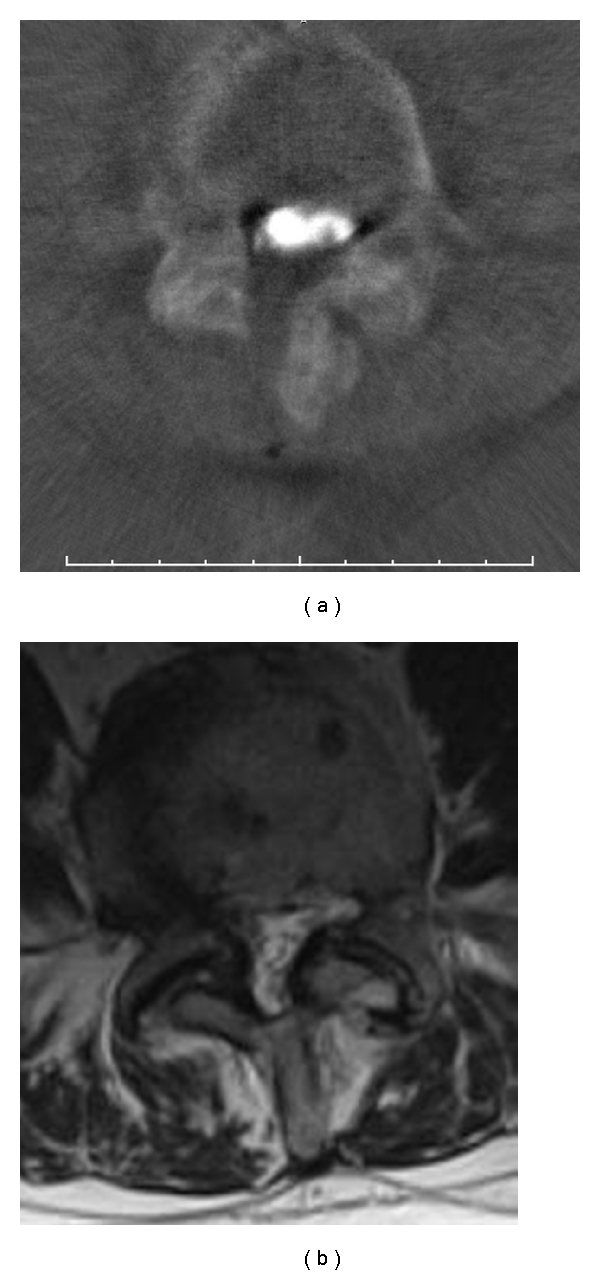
(a) Axial reconstruction with myelography demonstrating decompression from the left side with intraoperative myelography and insufficient decompression of the recess on the right side. (b) Preoperative MR image of the same patient as in (a).
